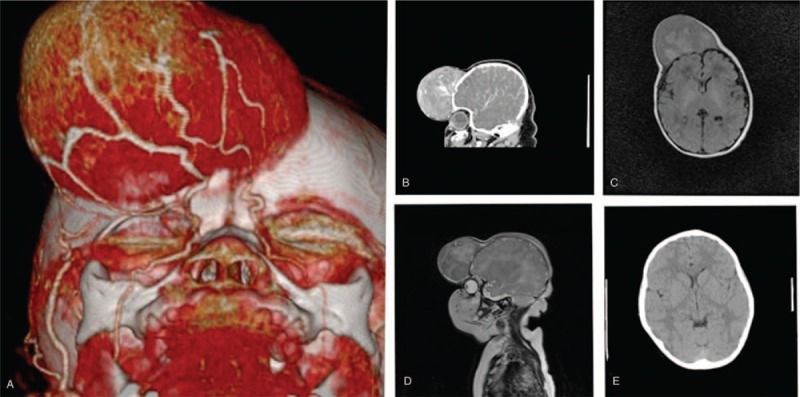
Multimodality imaging performed in this infant. (A) CTA demonstrated that the blood supply to the vascularized tumor originated mainly from the bilateral supratrochlear artery and partly from the distal part of the right superficial temporal artery. MRI showed a right extracranial mass with heterogeneous intensity on contrast-enhanced CT (B) and isointensity on T1-weighted (C) and T2-weighted images (D). There were no signs of recurrence or metastasis of the neoplasm on a CT scan (E).
